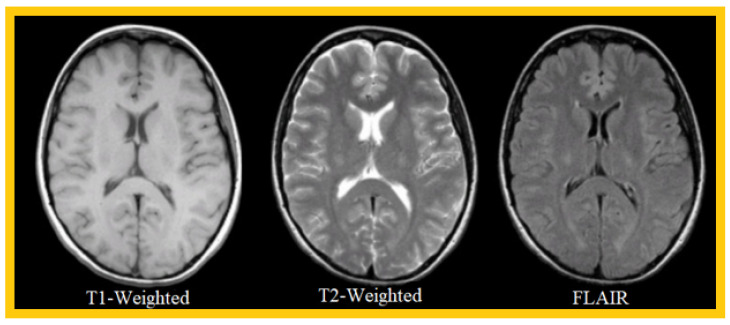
Example of 3 MRI sequences: T1-Weighted, T2-Weighted, and FLAIR.
